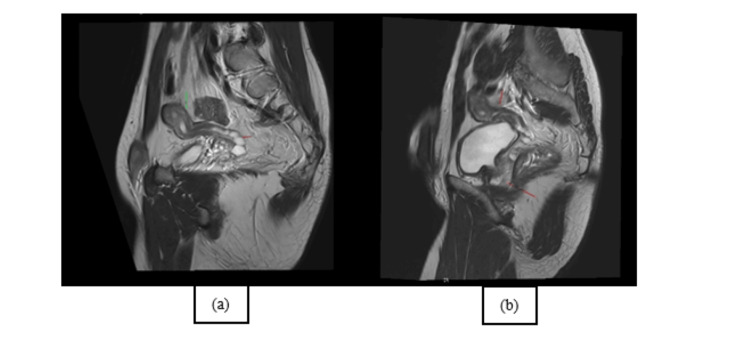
Sagittal T2-weighted image (a) and sagittal oblique T2-weighted image (b) show the zonal anatomy with the endometrium, which is hyperintense; the junctional zone as a hypointense band; and the outer myometrium with an intermediate signal. Also, on the sagittal T2-weighted image (a), there are also multiple cystic-like round nodules compatible with seminal vesicles; the sagittal oblique T2-weighted image (b) demonstrates the normal prostate anatomy shown before on CT scans. This figure is the original work of the authors. Patient consent for the use of the image was obtained, as mentioned in the patient consent form.
